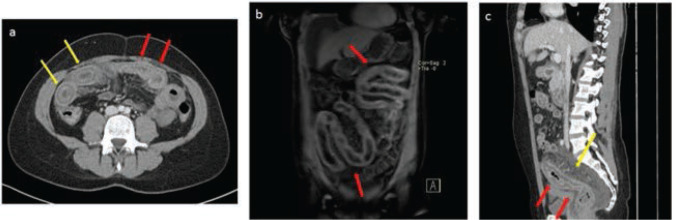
Abdominal CT, MR enteroclysis images of patient 4. (a) Axial CT image; jejunal wall thickening, target sign (red arrows); wall thickening of the ileum (yellow arrows). (b) MR enteroclysis image; wall thickening of jejunum and ileum (red arrows). (c) Lateral CT image; wall thickening of sigmoid and rectum (red arrows); ascites (yellow arrow).
